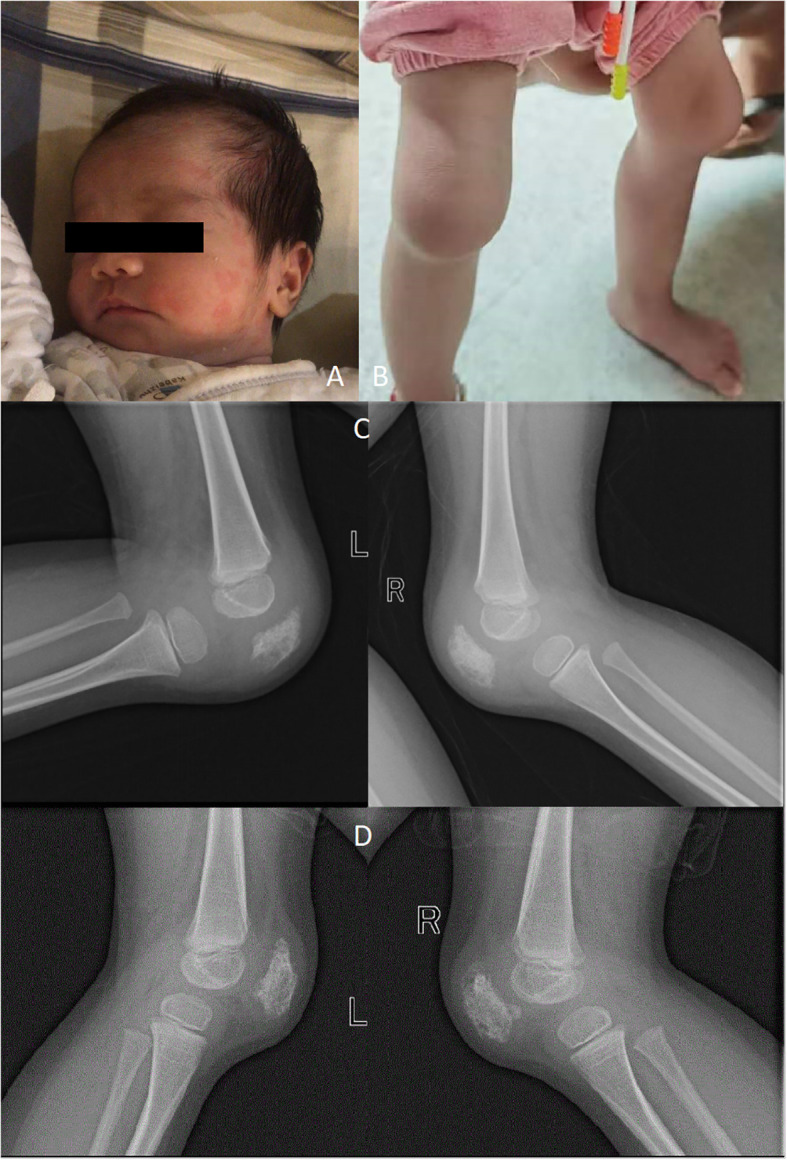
Rash (A); Deformities of the knee (B); X-ray showing destruction of patella (C); X-ray of the knee were taken half a year after Canakinumab treatment (D).
